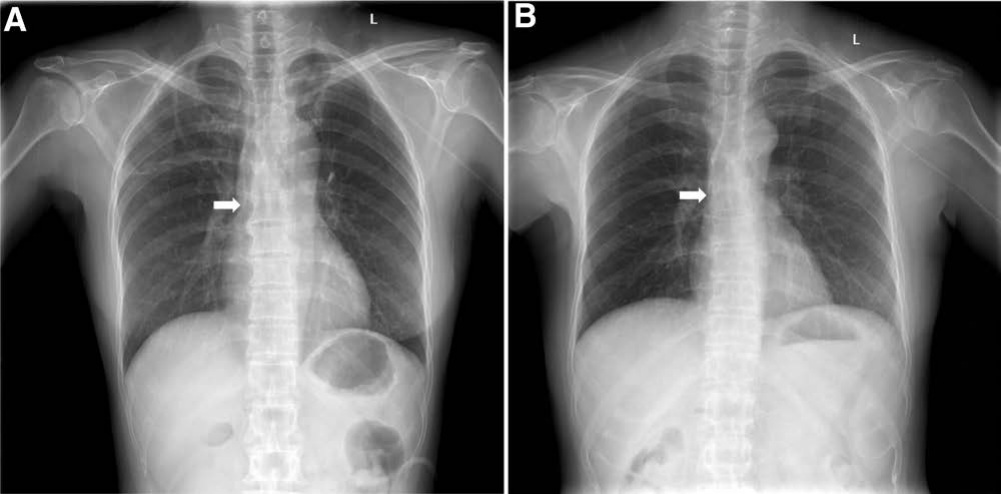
After the insertion of the PICC, Chest X-ray showed that the tip of the PICC was located at the lower third of the SVC (white arrows point to the tip of the PICC). (A) for patient A and (B) for patient B. PICC = peripherally inserted central catheter, SVC = superior vena cava.
